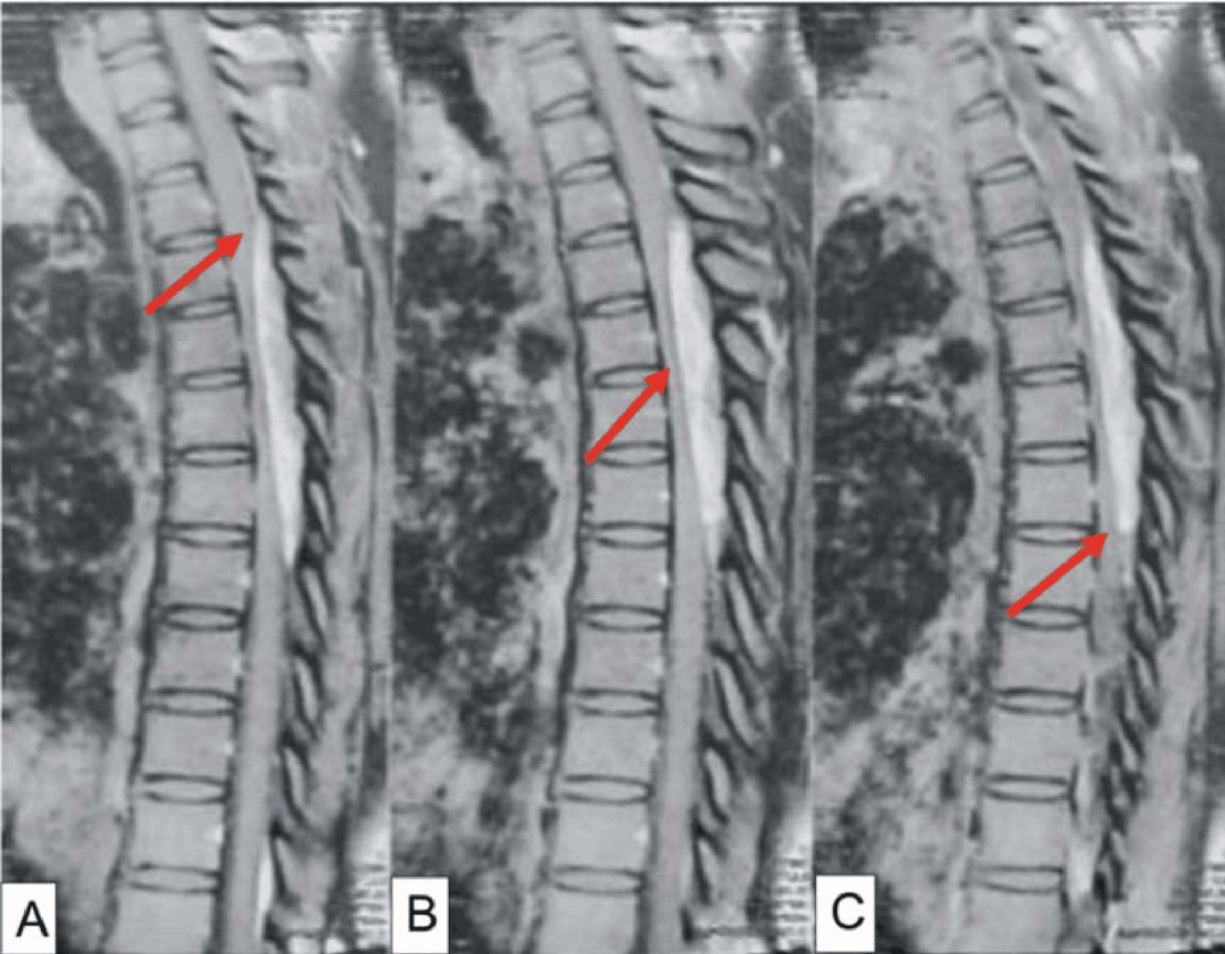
Dorsal MRI in T1 sagittal section showing the posterior epidural lesion (A) Dorsal MRI in T1 sagittal section showing the superior extent of the posterior epidural lesion. (B) Dorsal MRI in T1 sagittal section showing the posterior epidural lesion extension from D1 to D6, responsible for spinal cord compression. (C) Dorsal MRI in T1 sagittal section showing the inferior extent of the posterior epidural lesion
